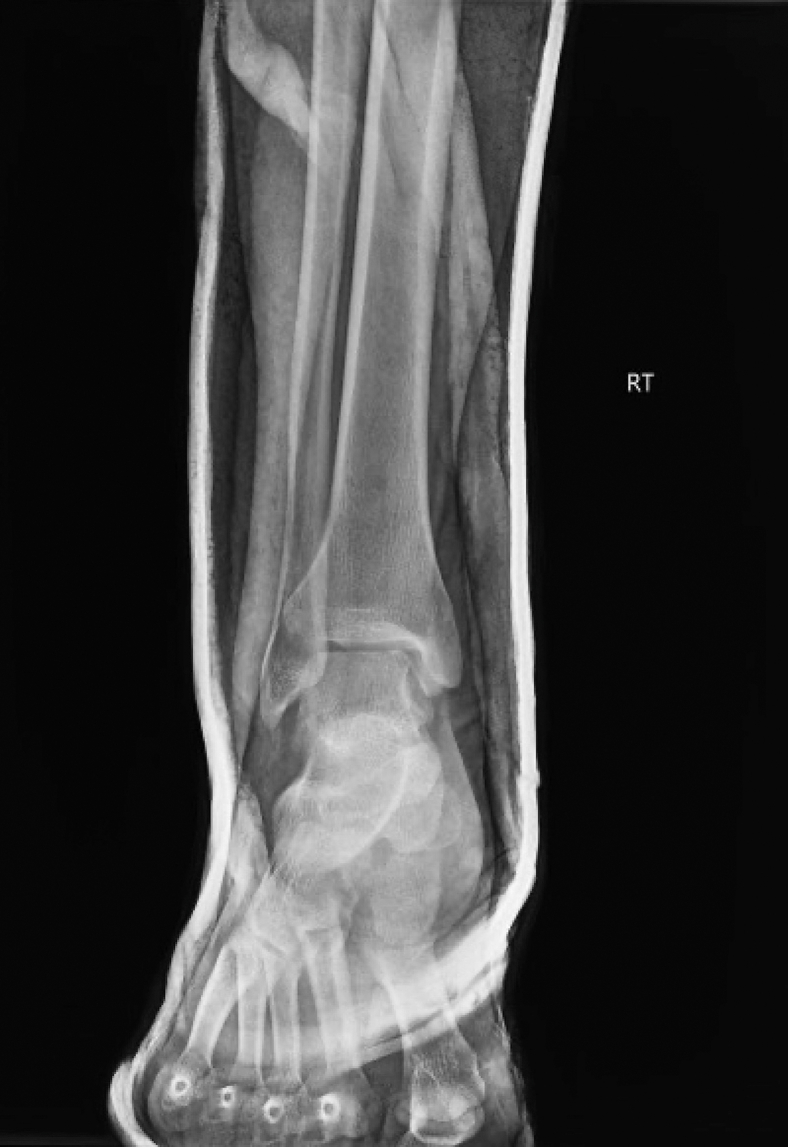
Postreduction, anteroposterior radiograph of the ankle showing closed reduction of a right-sided, posterior ankle dislocation.
